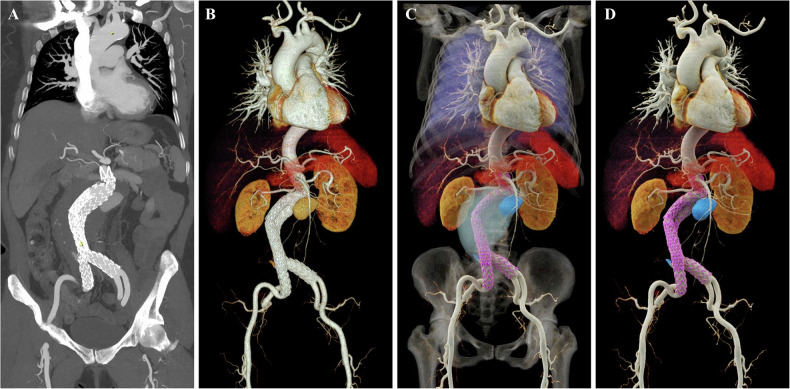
Follow-up images of a 61-year-old patient post-endovascular aneurysm repair (EVAR). A The CTA-based MIP image reveals stent shadows within the abdominal aorta and bilateral common iliac arteries, surrounded by slightly hypodense areas, with a visible localized endoleak. B The VR image visualizes only the contrast-enhanced portion of the endoleak, with non-enhanced regions poorly displayed. C, D CR images clearly depict the vessel wall and the mesh configuration of the metallic stent (purple). Utilizing high dynamic range rendering, these images distinctively differentiate between the surrounding chronic thrombus/organized vessel wall (light blue) and newly formed contrast-enhanced endoleak components (blue), precisely delineating the extent and source of the endoleak
